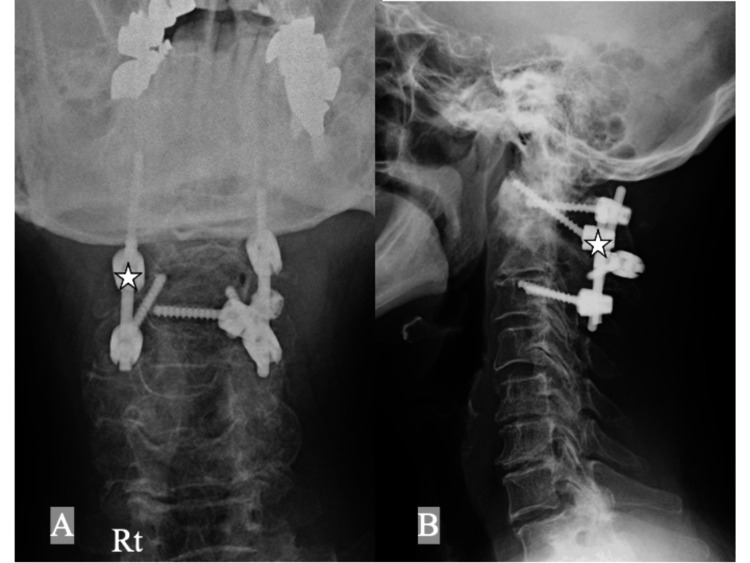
Postoperative cervical spine plain X-rays, (A) anteroposterior and (B) lateral views, of the cervical spine after surgery showing a C1-C3 posterior fusion with C1 lateral mass screw inserted from the caudal side of the C2 nerve root on the right (ponticulus posticus side, white stars) and via the posterior arch on the left (C1 pedicle screw), the right C2 laminar screw, and bilateral C3 pedicle screws.
